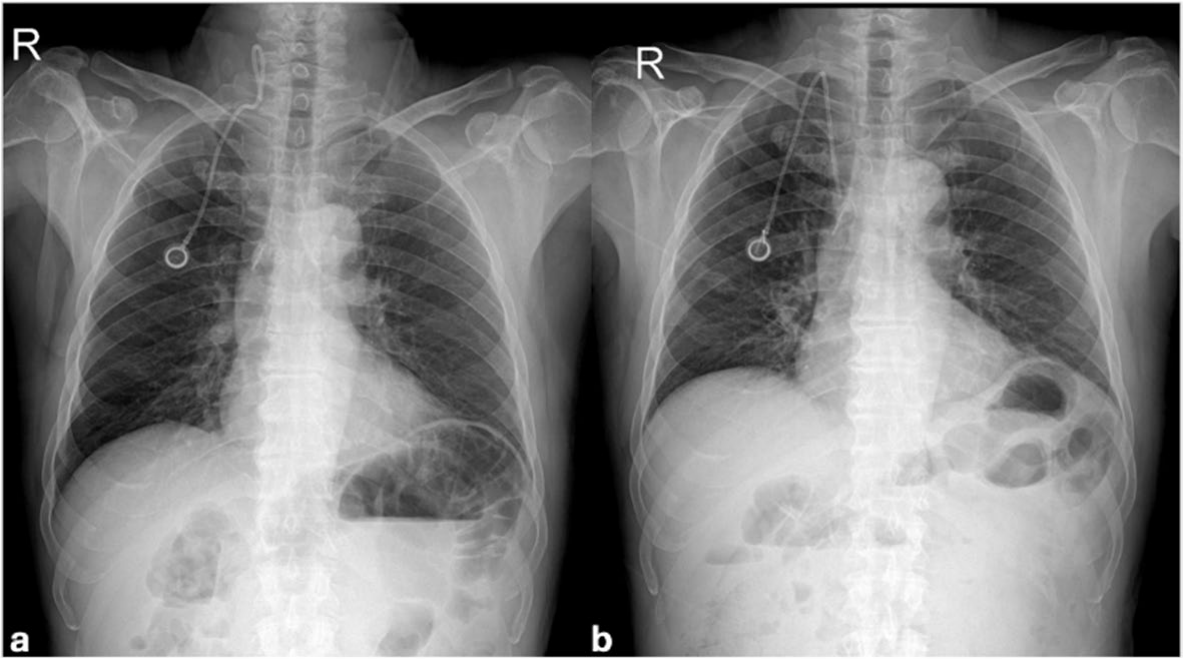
Chest radiography performed for port dysfunction showed that the port catheter was malpositioned in the right jugular vein (a, July 2024). The patient's last available radiological study showed that the catheter was in the superior vena cava (b, May 2024)
